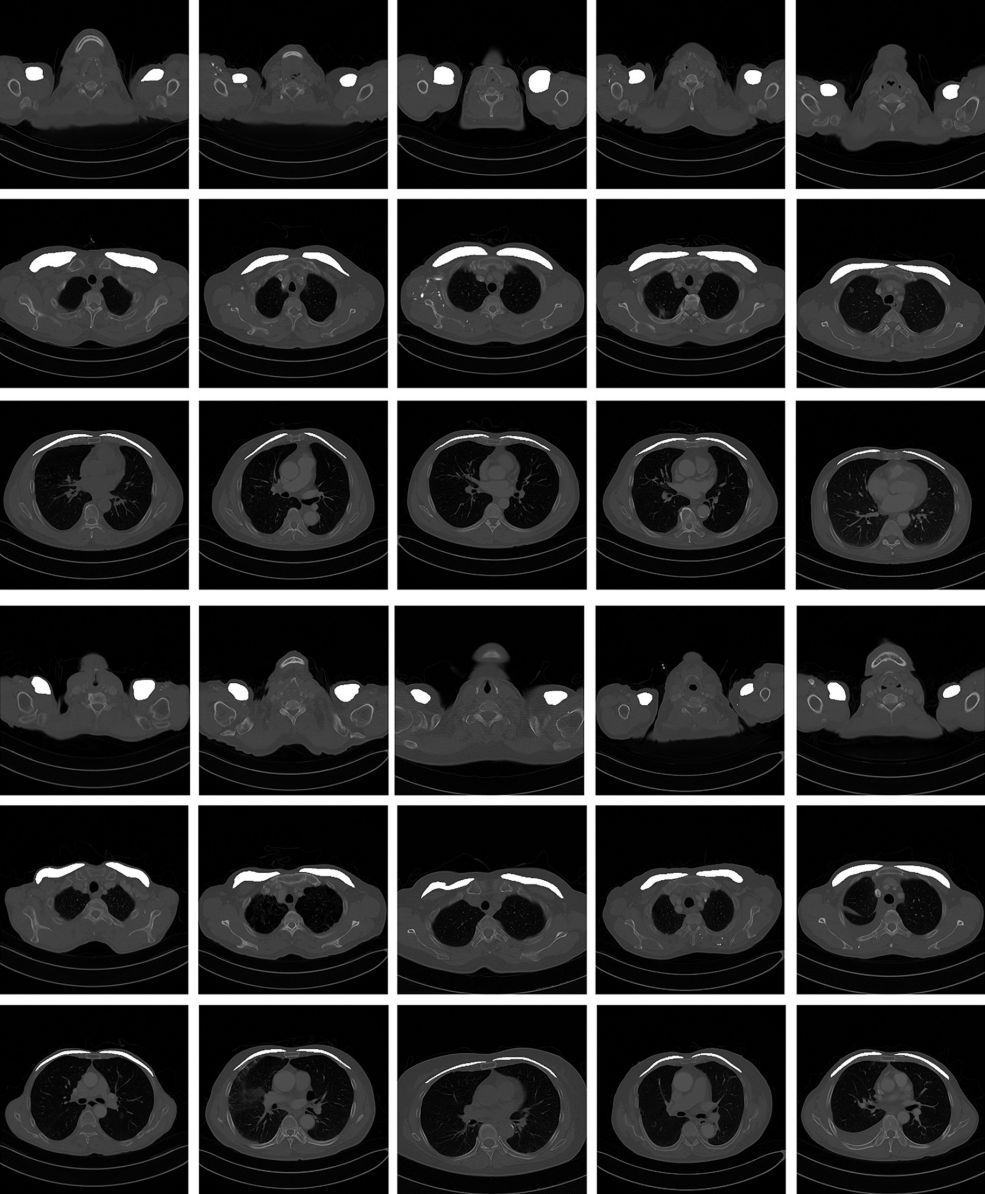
Sample results of the predicted pectoralis muscle area from the patient CT image dataset.
